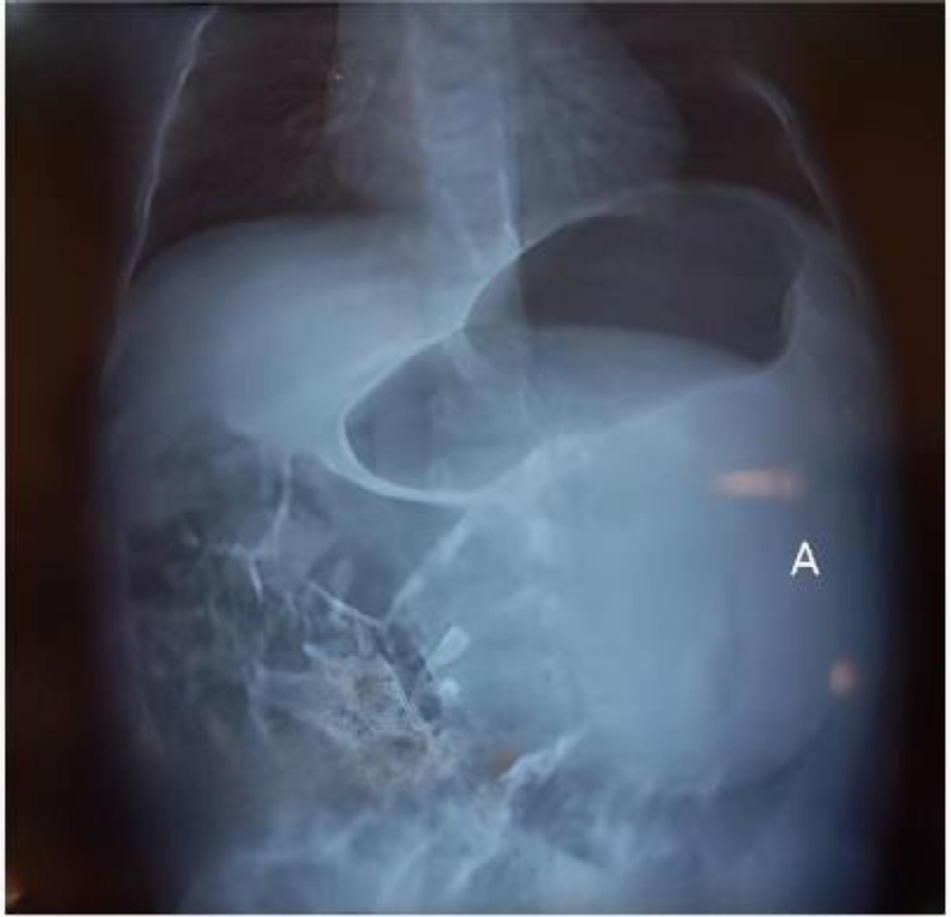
Frontal X-ray of a skeletal immature child. (A) demonstrating a predominantly cystic mass in the left hemi-abdomen.
